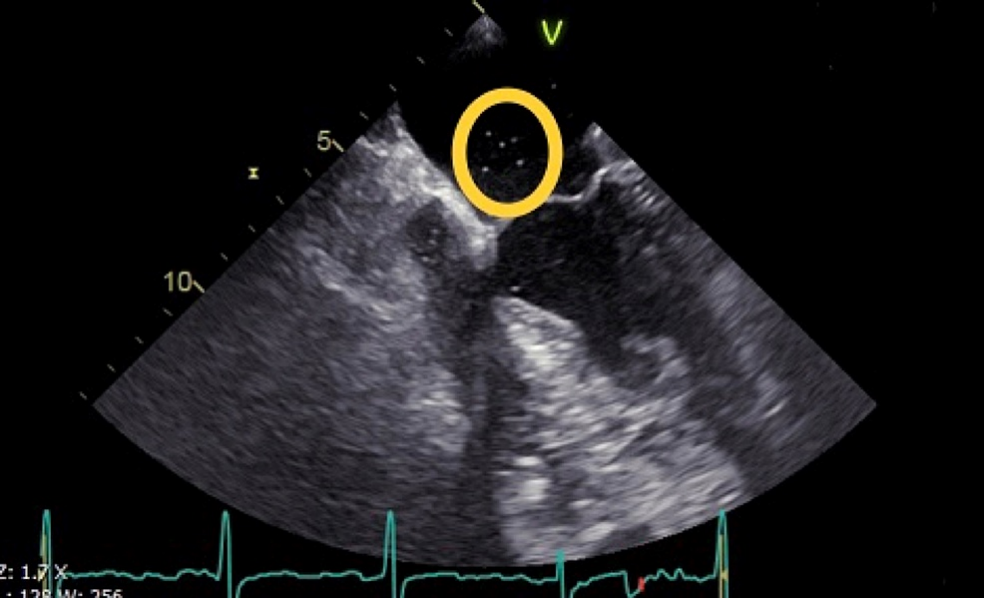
Transesophageal Echocardiogram (TEE) with yellow circle showing the RLIAS with evidence of PFO RLIAS: right-to-left interatrial shunt; PFO: patent foramen ovale
